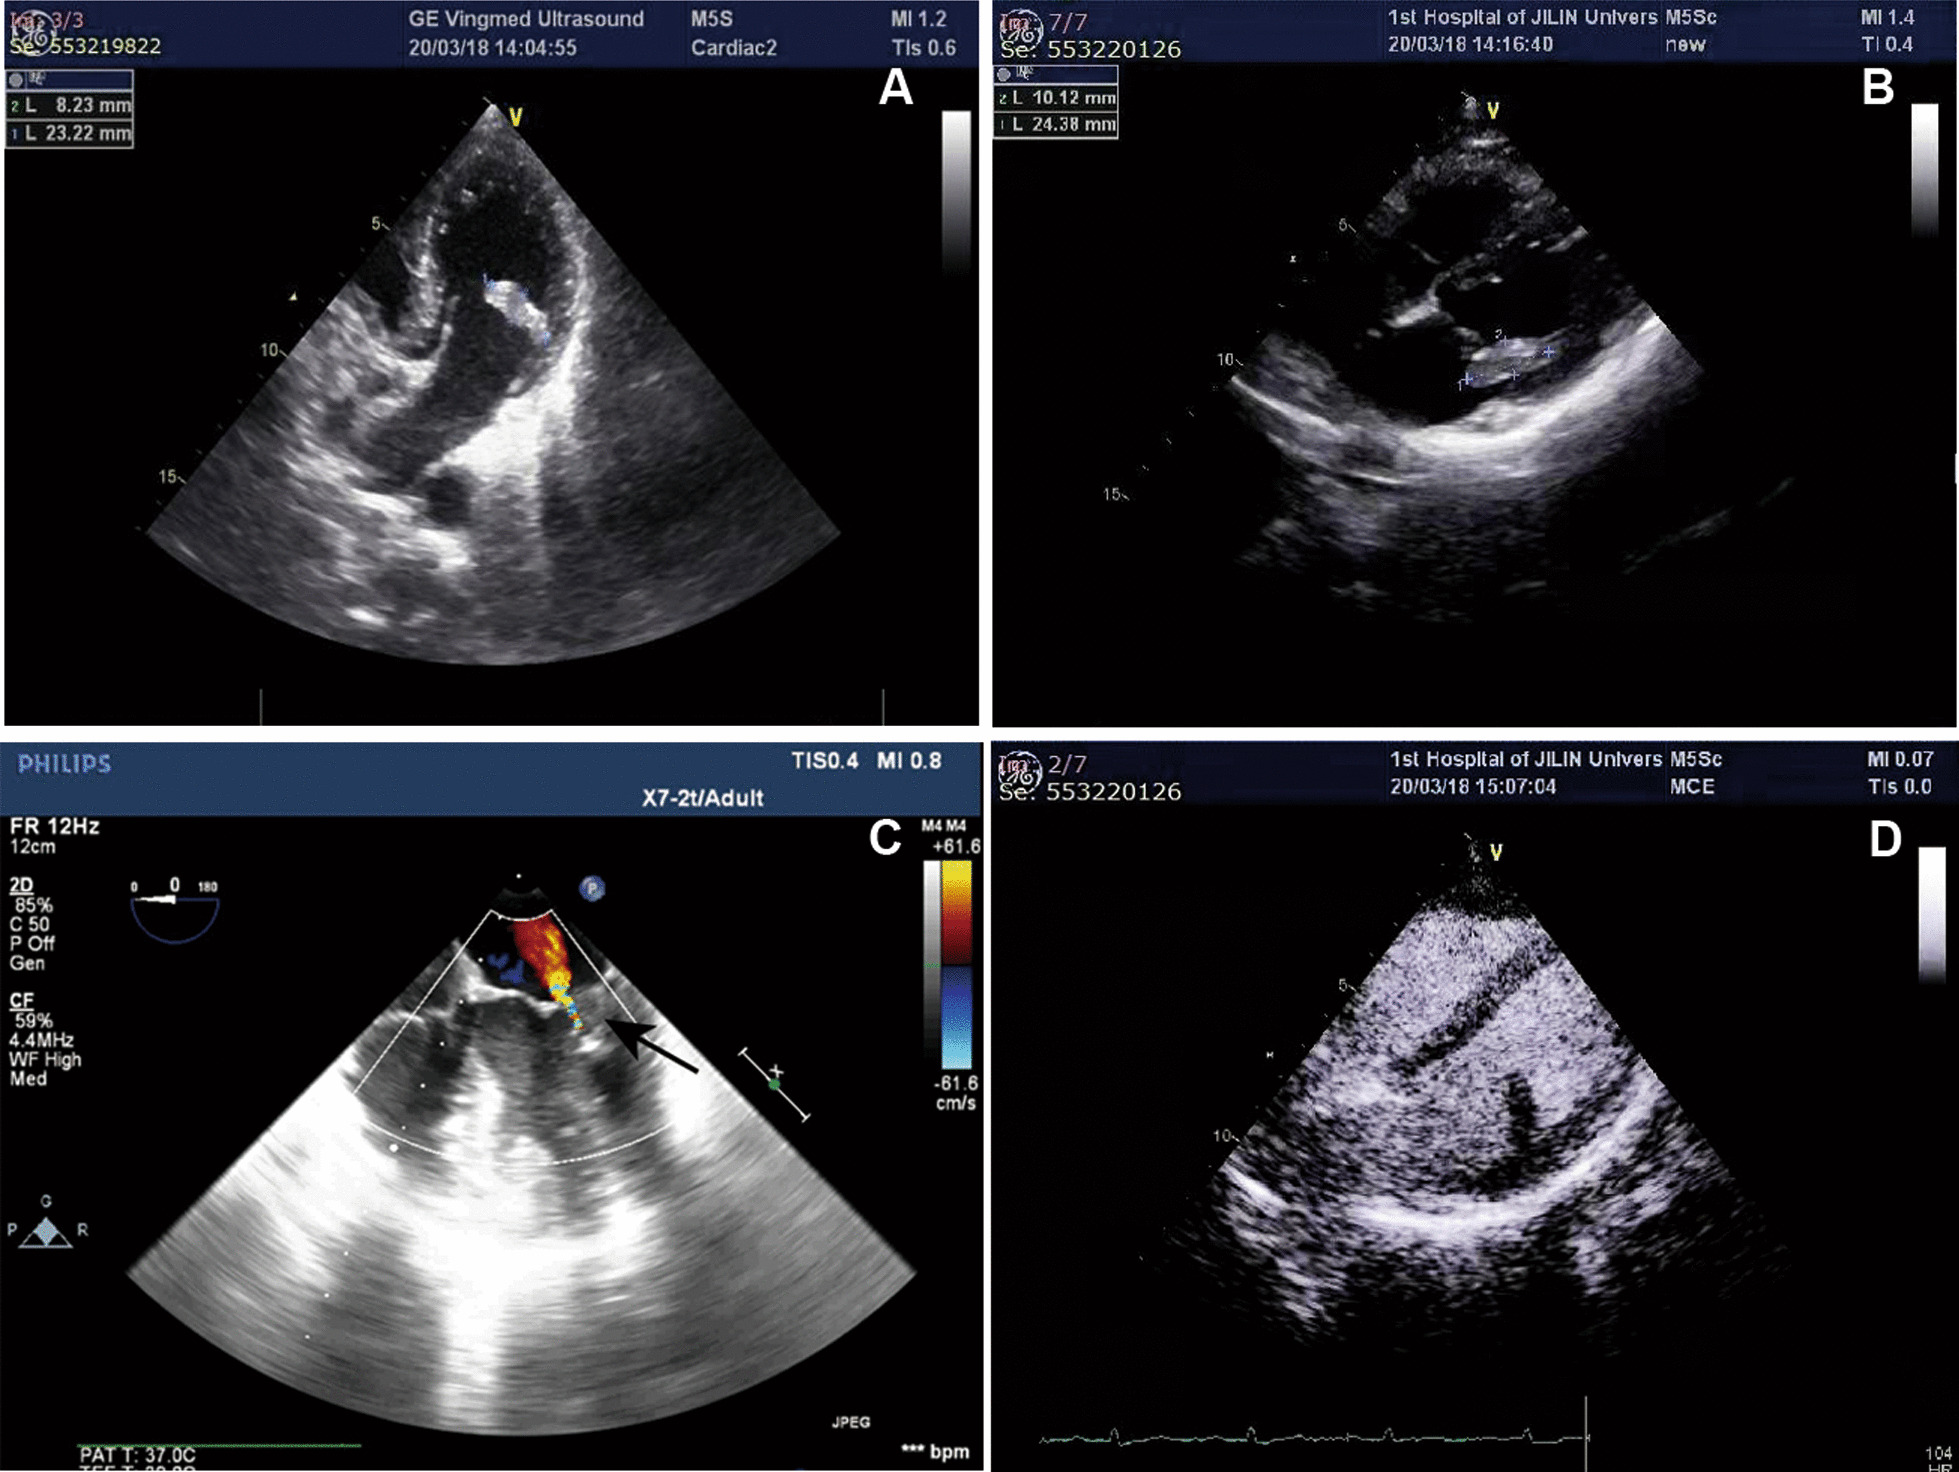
Ultrasound of the cardiac tumor. A Two-chamber view in transthoracic echocardiography shows a 23 mm × 8 mm mass which was polyp-like-shaped, homogeneous, firm, mobile and solitary centered on the lateral wall of the left ventricle. B Grey and C Color doppler flow imaging of four-chamber view in transesophageal echocardiography shows that the mass had regular margins and was pedunculated, with a stalk originating from the LV wall, and the mass protruded into the left atrium during systole, resulting in mild mitral regurgitation. D View of left ventricular contrast-enhanced echocardiogram shows little contrast agent filling
